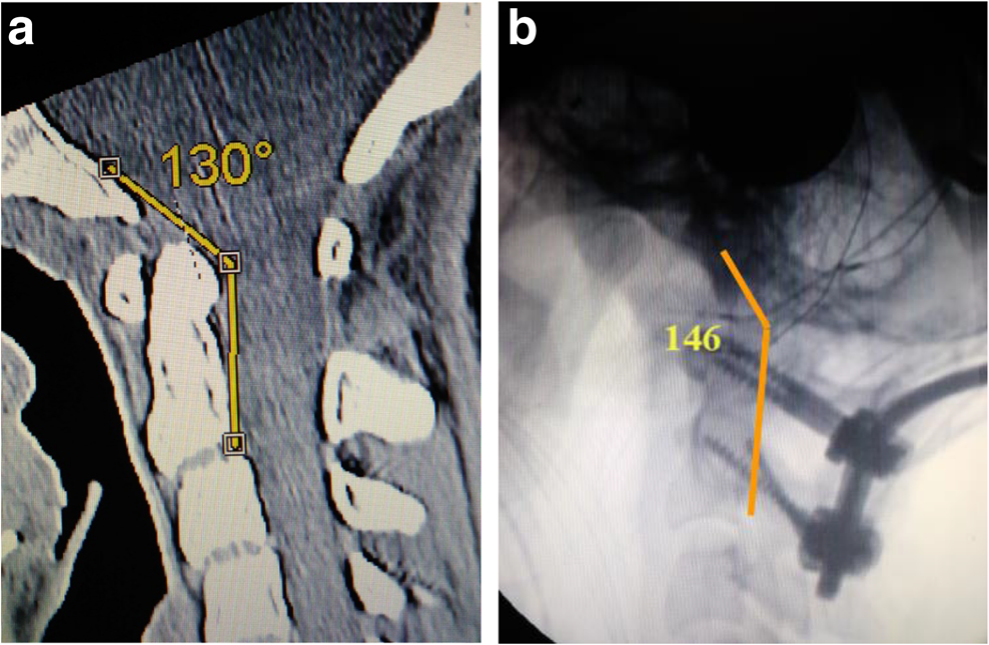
Intraoperative reduction: the preoperative CT (i) shows a CXA of 130°; the intra-operative fluoroscopic image after reduction (ii) shows a CXA of 146°
